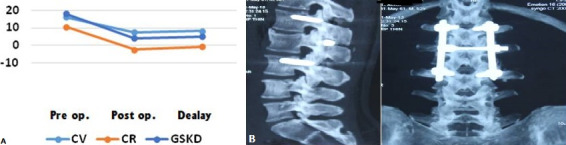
A) evolution of the radiological parameters; B) CT images in favor of the outline of anterior arthrodesis
